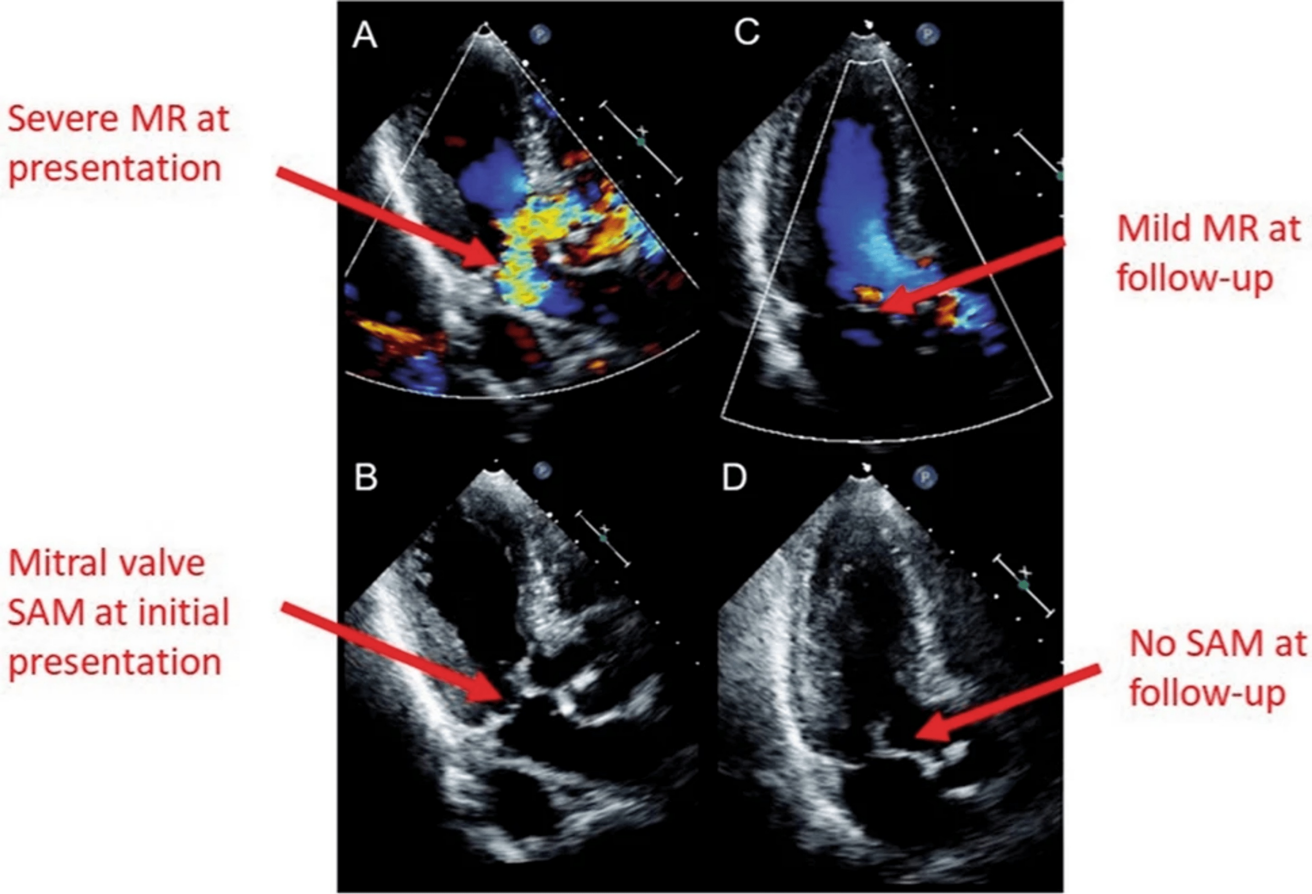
Two-dimensional transthoracic echocardiography, apical long-axis views, in patients with mitral regurgitation (MR) due to systolic anterior motion (SAM) of the mitral valve. (A) Severe MR at initial presentation. (B) SAM of the mitral valve at initial presentation. (C) Only mild MR was found at follow-up. (D) SAM of the mitral valve was not found at follow-up [10].
